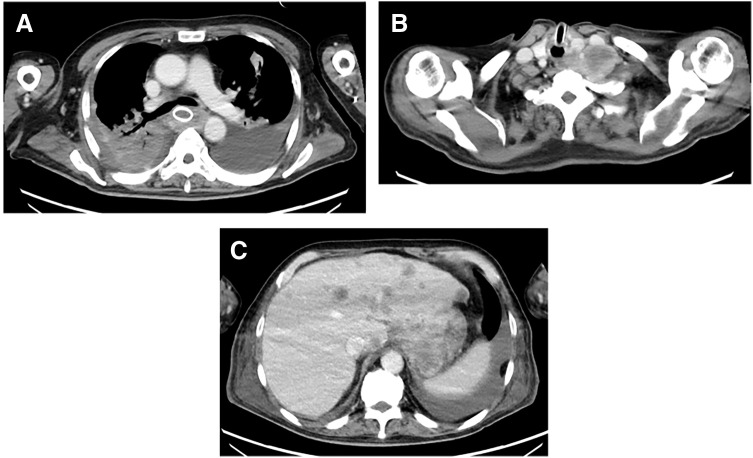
Follow-up CT demonstrating rapid disease progression. (A) Axial CT shows progressive left supraclavicular nodal disease. (B) Axial CT demonstrates bilateral pleural effusions/pleural disease after recovery from the acute septic episode. (C) Axial CT shows multiple liver metastases, consistent with rapid systemic progression.
